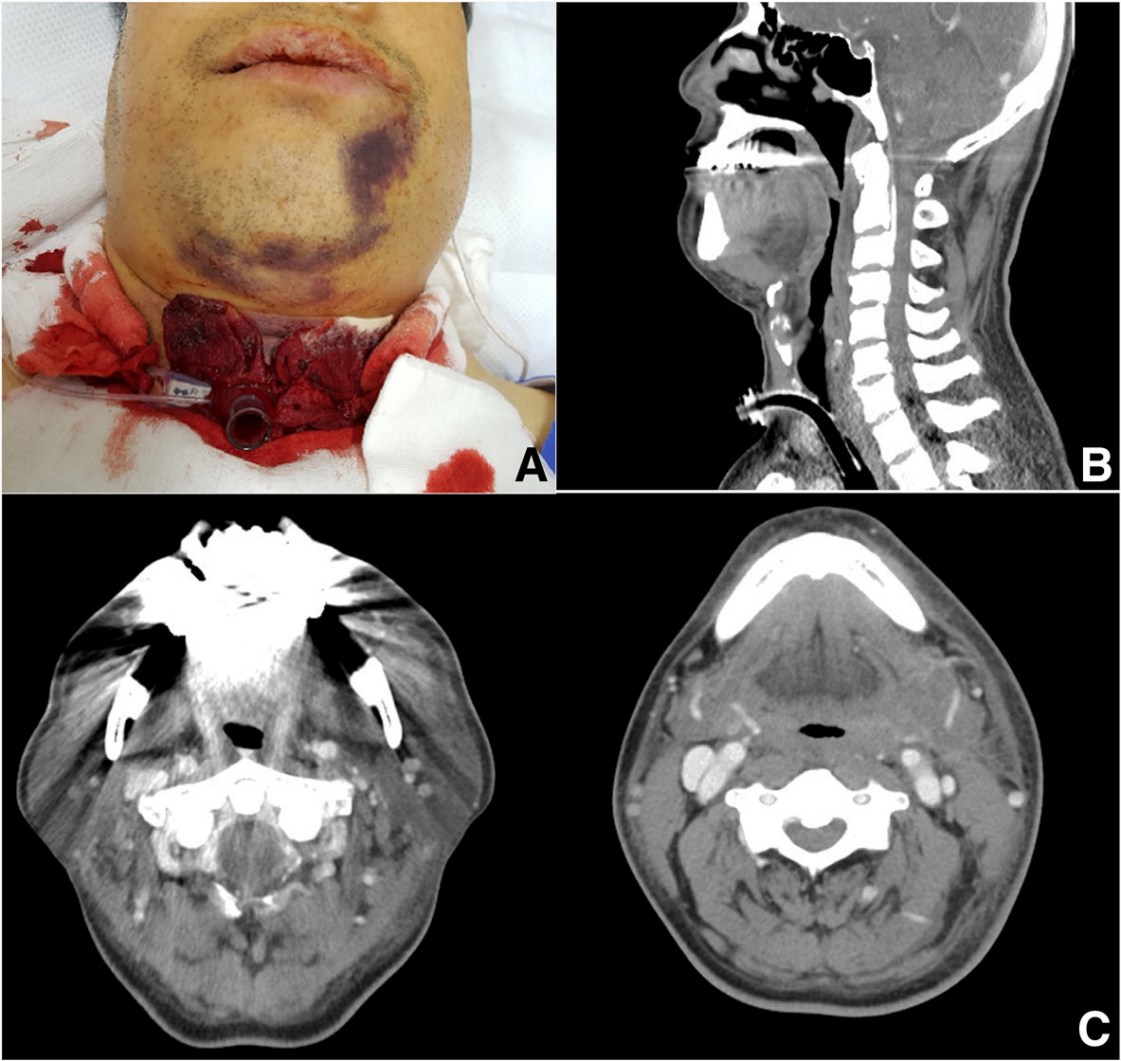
a Constant bleeding at the tracheostomy site after an emergency tracheostomy. b Follow-up sagittal CT view and c axial CT views after 3 days showed decreased soft tissue swelling along the superficial and deep neck spaces at 3 days after tracheostomy
